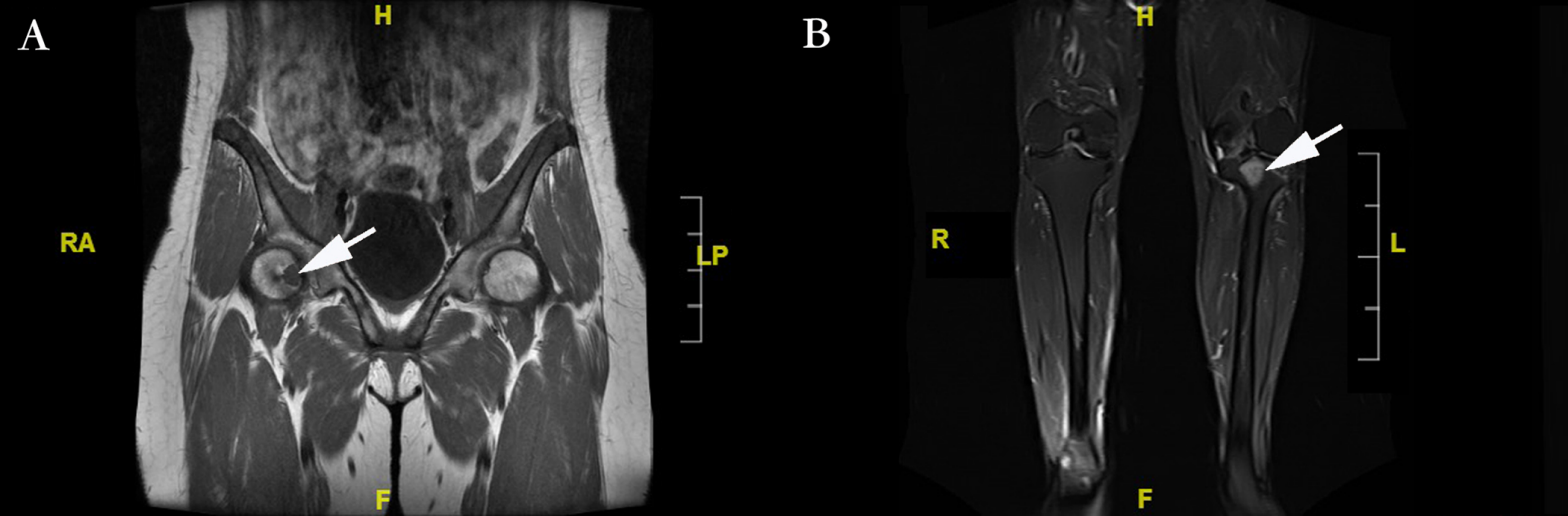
The causative lesion of TIO, as shown by MRI to identify the particular location. 1A. A 24-year-old female patient with tumor-induced osteomalacia has a lesion located in the right femoral head. The arrow represents the tumor area. 1B: Causative lesion located in the tibial plateau of a 51-year-old male tumor-induced osteomalacia patient. The white arrow represents the tumor area.
